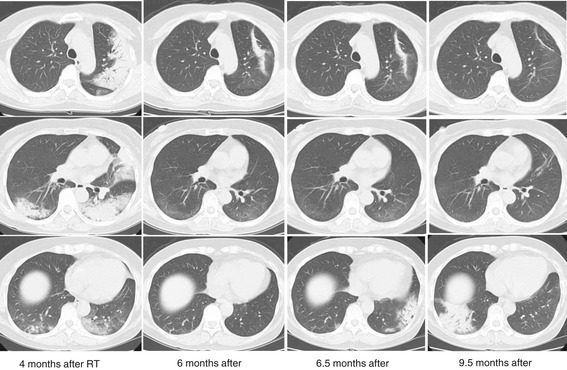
55-year-old patient received irradiation in the whole left breast (50 Gy/25 fractions) with an electron boost (10 Gy/5 fractions) to the partial breast. The lung V20 was 6.0%. Four months after RT following breast-conserving surgery, a severe cough, fever and decreased oxygen saturation measured by a pulseoximeter (SpO2; 88% at room air) were observed. Pulmonary lesions outside the irradiated field were observed on chest radiographs, which were located in the bilateral lungs on CT. Prednisolone was started at 30 mg/day based on a diagnosis of BOOP syndrome. The prednisolone was gradually tapered off, because the symptoms and pulmonary lesions were improved. However, the pulmonary lesions appeared again at 6.5 months after RT without any symptoms. Prednisolone was again administered until 7.5 months after RT. Although the pulmonary lesions appeared again at 9.5 months after RT, there were no symptoms.
